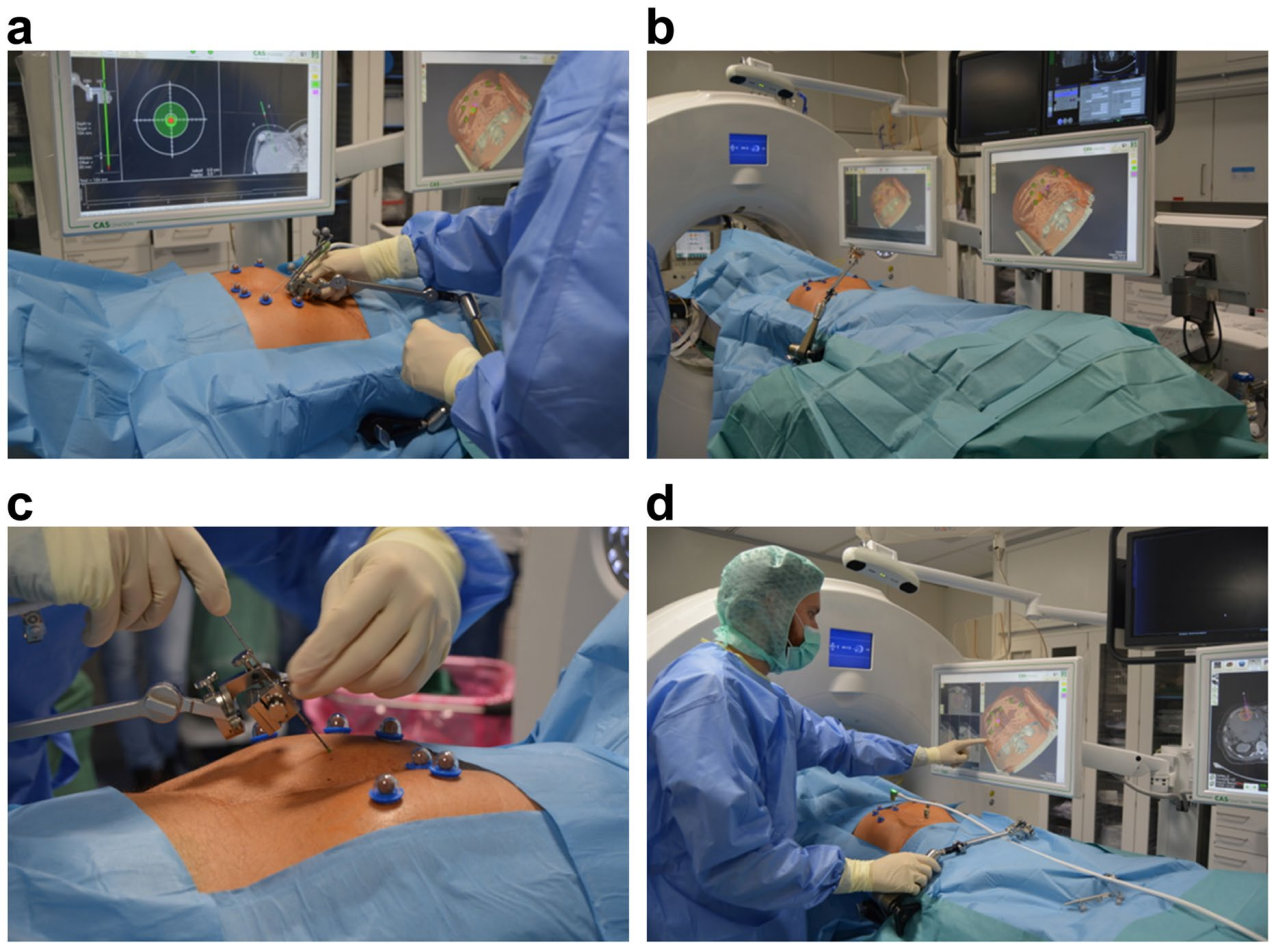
Setup and operation of the navigation system for ablation of malignant liver tumors. (a) A precise setting of the aiming device is crucial for optimal probe positioning. It is important that the positioning is always performed in apnoea, otherwise, major deviations must be expected. (b) Device setting and patient positioning: The computed tomography (CT)- and navigation-device-monitors are placed on the opposite side of the specialist. The tracking camera must be freely positioned to ensure optimal navigation. The aiming device is fixed on the side of the specialist. (c) The probe is fixed in the provided holder and the fine adjustment is made before puncturing the skin. It is important to place the optical markers outside the stitch area. (d) One of the two monitors should be covered in sterile material so that the specialist can check and readjust the optimal probe position directly.
